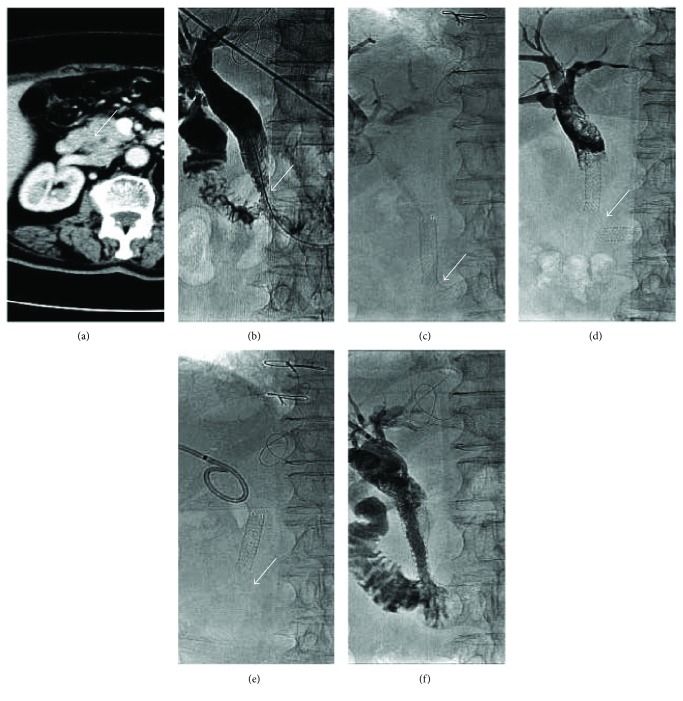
Gradual fracture of a biliary stent in a 78-year-old woman with common bile duct obstruction secondary to carcinoma of the ampulla of Vater. (a). Abdominal-enhanced CT scan showing a mass located at the duodenal ampulla of Vater (arrow) was shown. (b). Percutaneous transhepatic cholangiogram showing severe stricture of the distal common bile duct was shown, and a SEMS (diameter, 8 mm; length, 60 mm; COOK Inc., Bloomington, IN, United States) was inserted to relieve the obstruction. (c)–(e). 249 days after SEMS insertion, percutaneous transhepatic cholangiography showed stent fracture located at the stricture position, from partial, filamentous connective to complete fracture, without the distal part of the stent (arrow). (f). A new SEMS (diameter, 8 mm; length, 80 mm; COOK Inc., Bloomington, IN, United States) was inserted to relieve the reobstruction.
